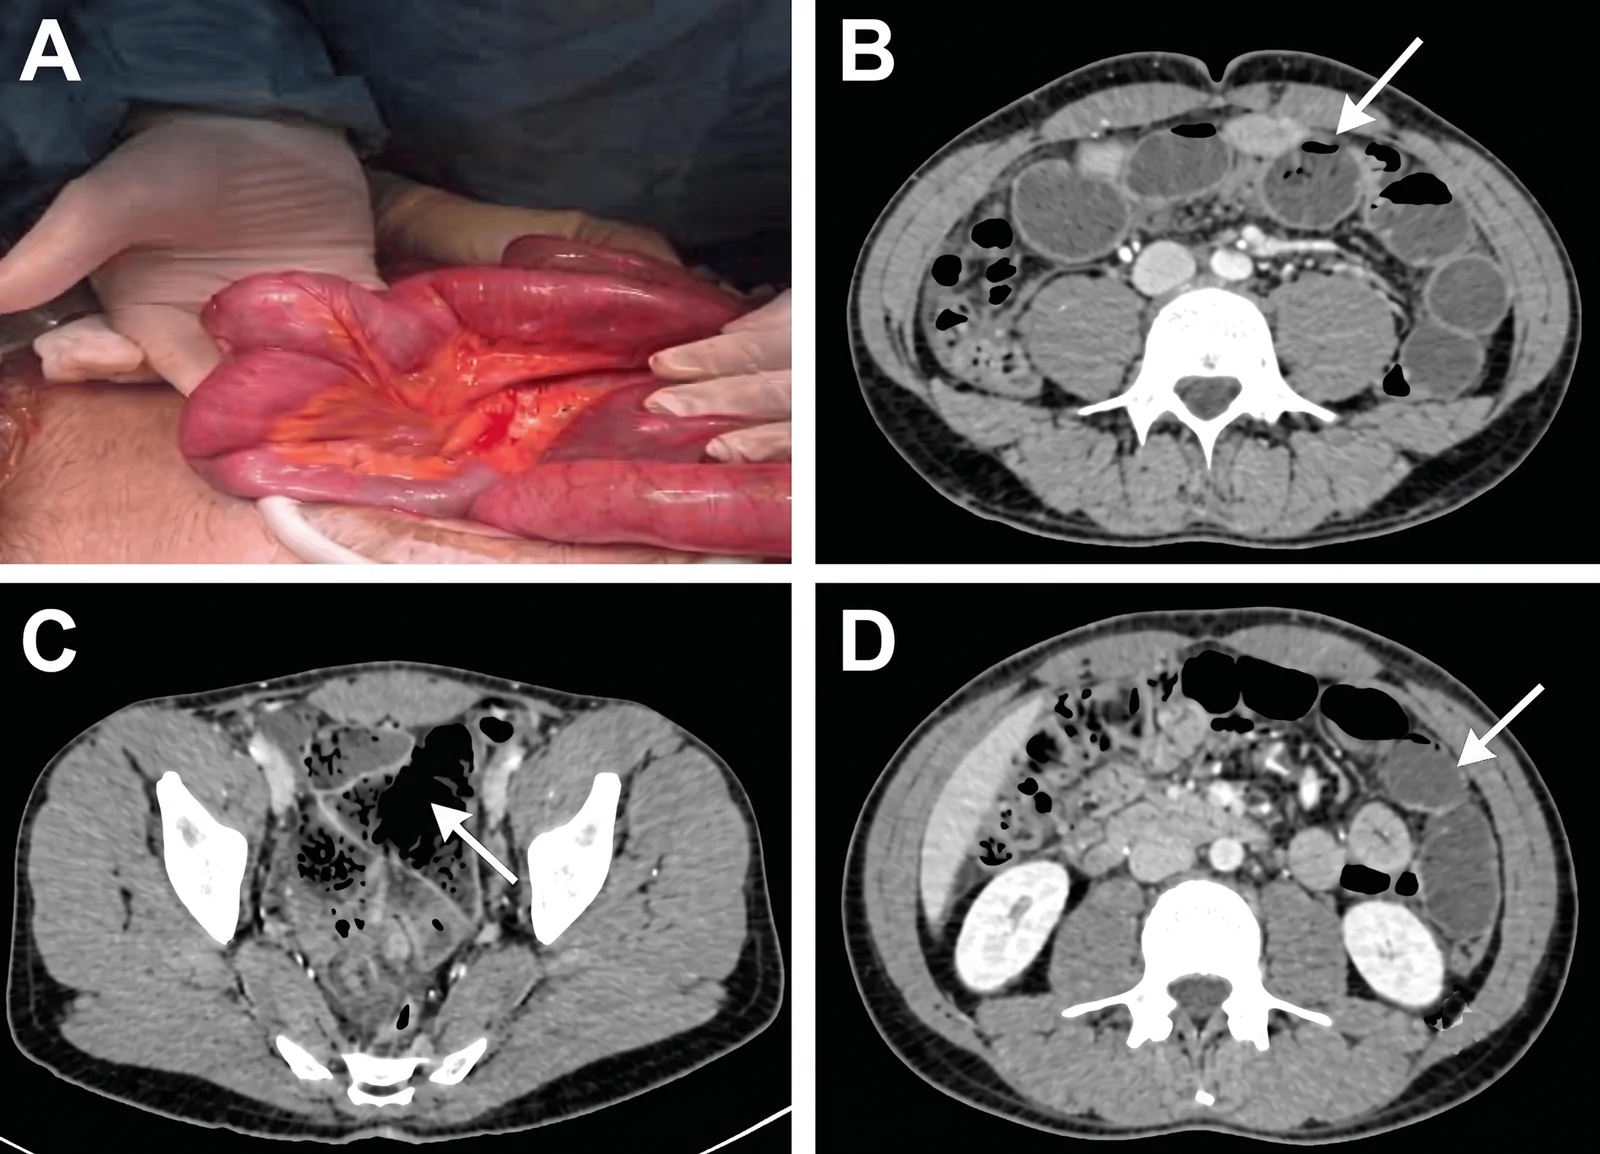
Radiological and intraoperative findings of phytobezoar-induced small bowel obstruction secondary to Meckel's diverticulum (A) Intraoperative photograph demonstrating dilated ileal loops at the site of obstruction caused by a phytobezoar impacted within a Meckel's diverticulum. (B) Axial contrast-enhanced CT image showing multiple dilated proximal small bowel loops (arrow) consistent with mechanical small bowel obstruction. (C) Axial CT image demonstrating the transition point in the distal ileum with the characteristic small bowel feces sign (arrow), represented by particulate intraluminal material intermixed with gas bubbles proximal to the obstruction. (D) Axial contrast-enhanced CT image demonstrating dilated proximal bowel loops (arrow) with decompressed distal bowel, confirming distal mechanical small bowel obstruction. CT: computed tomography
